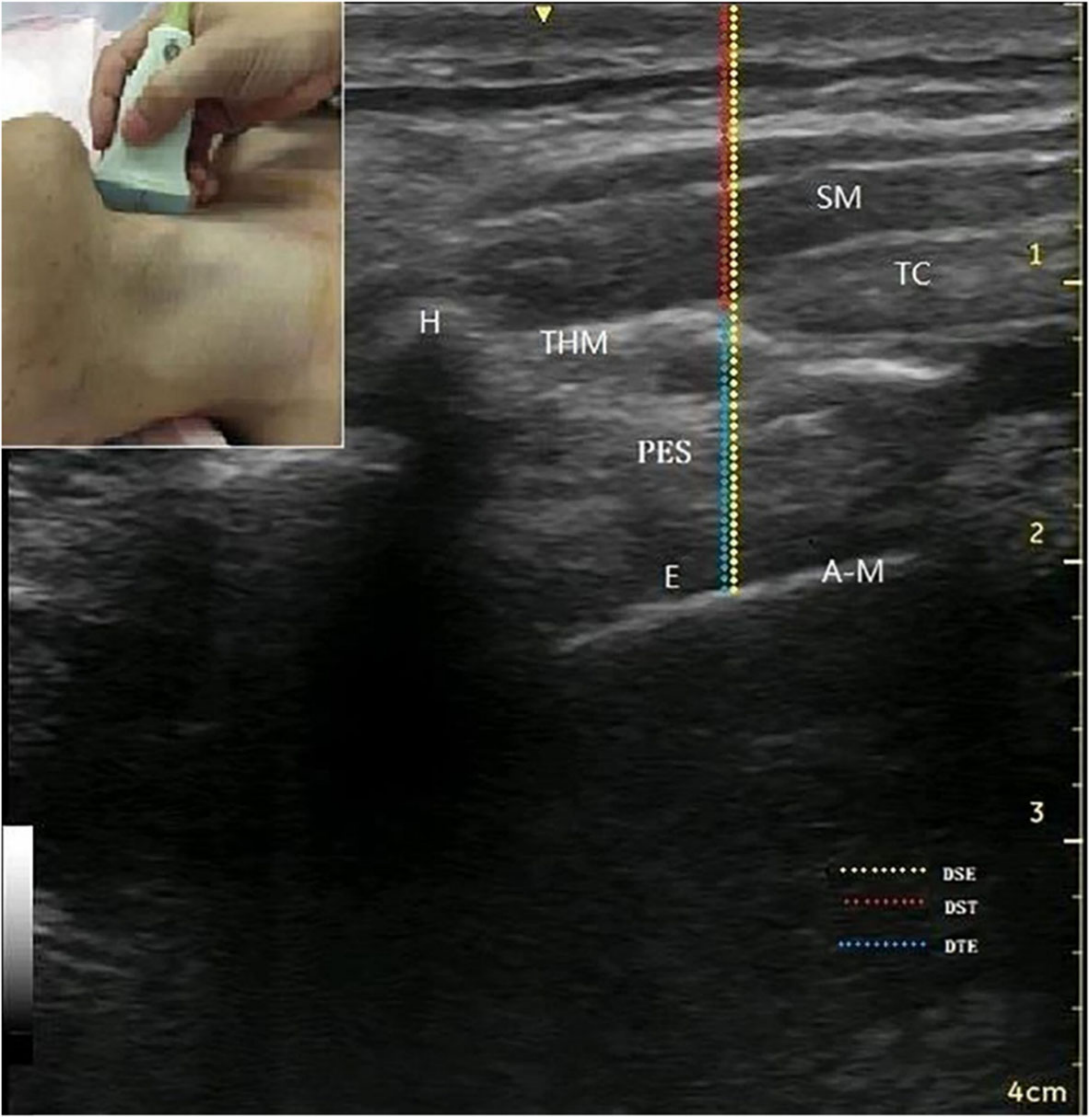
The parasagittal ultrasound view of laryngeal structures. H: hyoid; TC: thyroid cartilage; E: epiglottis; A-M: junction of air and mucous membranes; SM: strap muscles; DSE: distance between skin and epiglottis; DST: distance between skin and thyroid cartilage; DTE: distance between thyroid cartilage and epiglottis; PES: pre-epiglottis space
